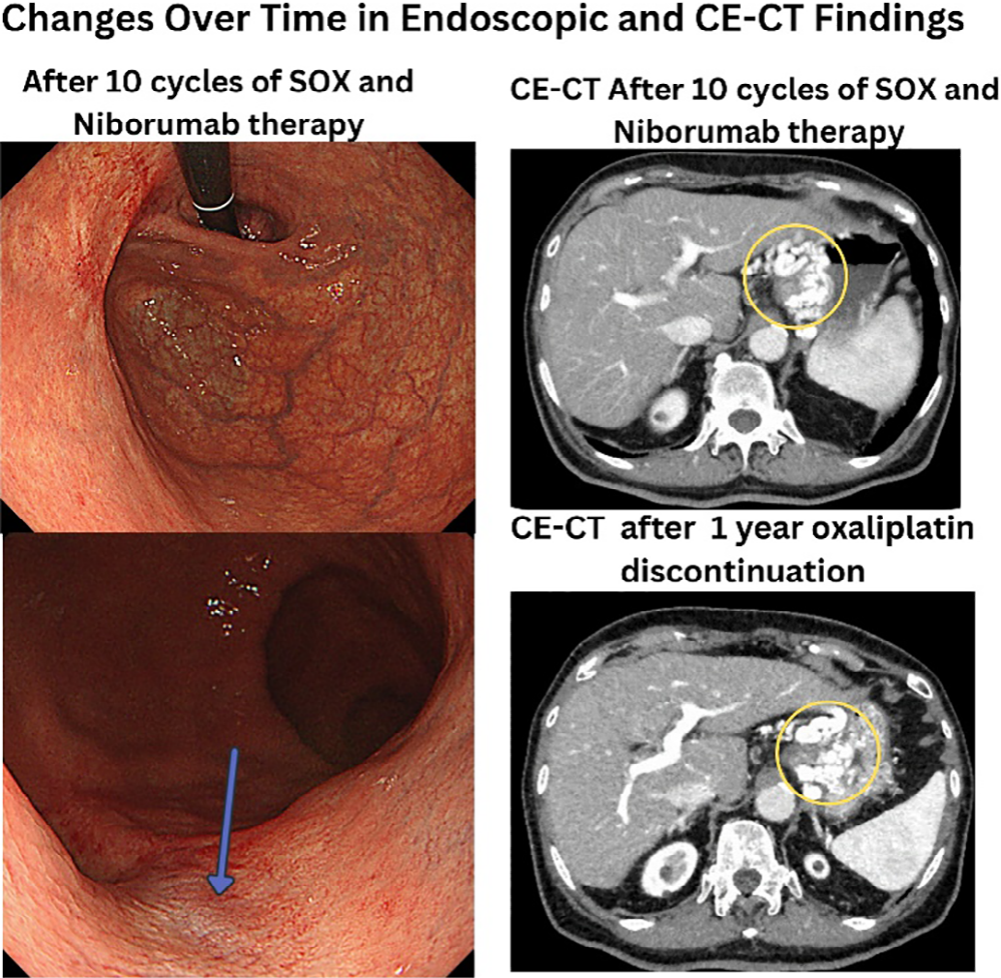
Endoscopic findings suspicious for the variceal‐like vessel (arrow), and complete response to chemotherapy. Contrast medium‐enhanced computed tomography (CE‐CT) shows a complete response to chemotherapy and the formation of a portosystemic shunt (circle) and suspected mild enlargement of the shunt 1 year after oxaliplatin discontinuation.
